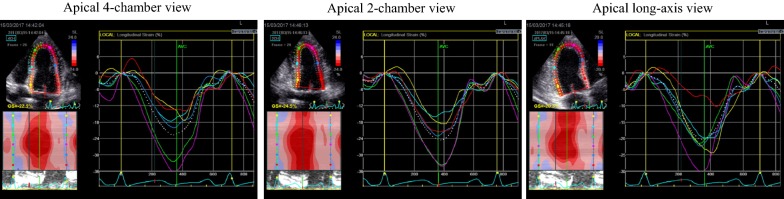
An example of a color-coded two-dimensional speckle tracking echocardiography (2D-STE) of the left ventricle (LV) and corresponding time–strain curves from 18 LV segments derived from the apical 4-, 2-chamber and long-axis views for measurement of global longitudinal strain (GLS) in diabetic patient with acute hyperglycemia. GLS was calculated as the average peak strain of 18 LV segments, and in this example the value of GLS is − 22.4%
